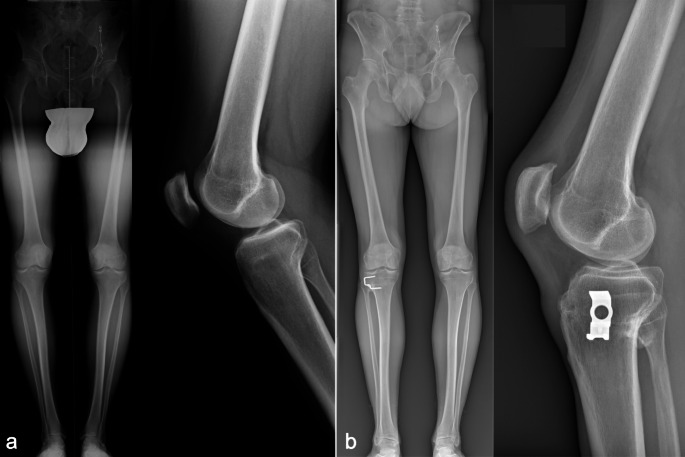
Case example: (a) preoperative full length weight bearing and lateral view radiograph; (b) full length weight bearing and lateral view radiographs at nine years of follow up
